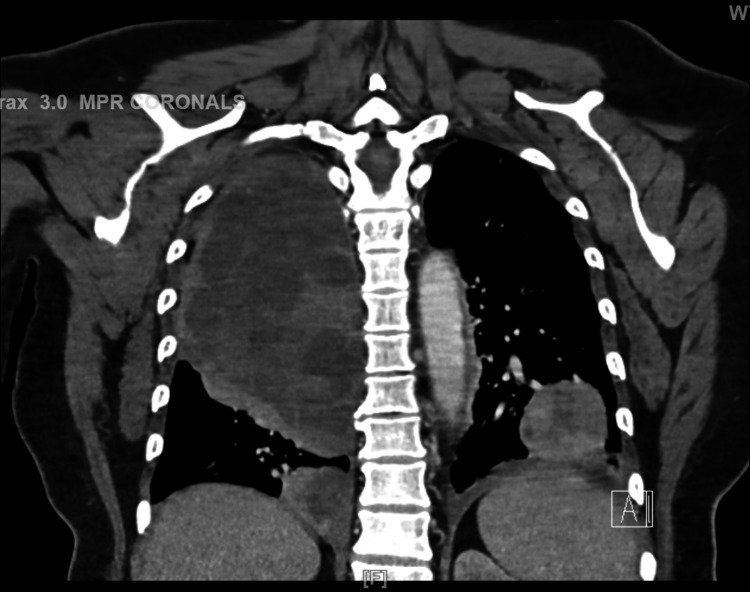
Coronal view of CT chest
